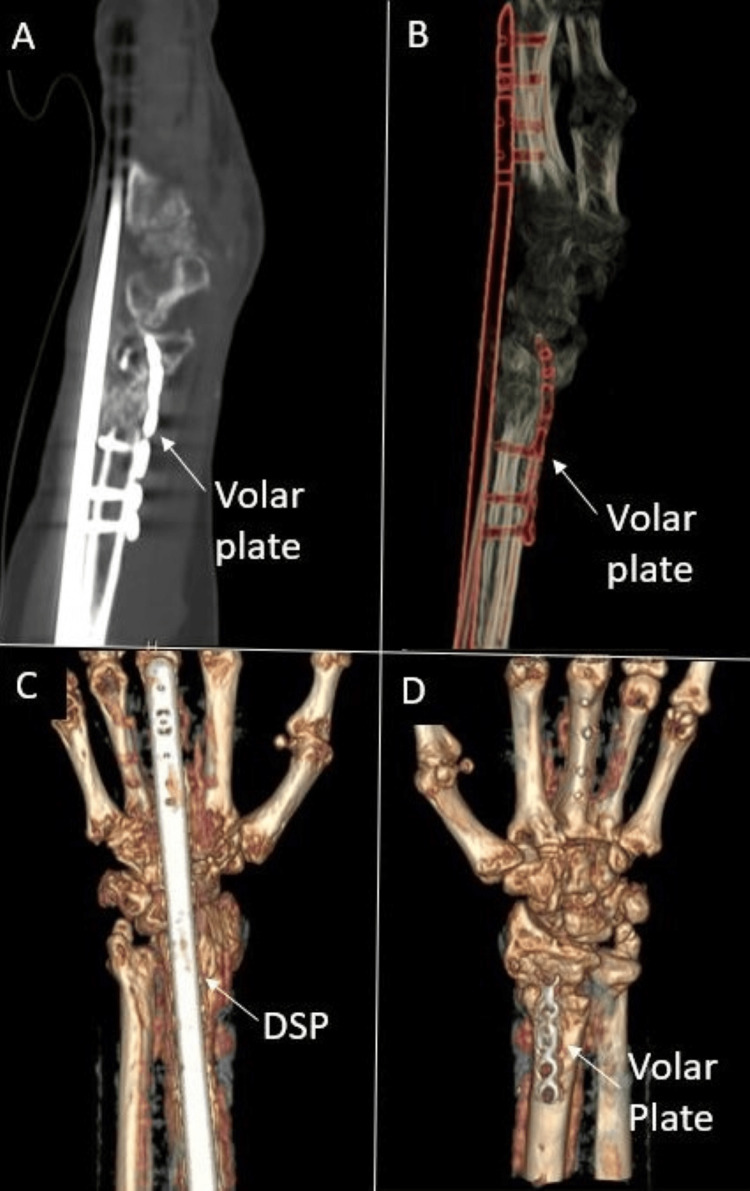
CT left wrist two months status post second fixation A/B: CT films demonstrating dorsal spanning plate (DSP) and volar intramedullary fragment plate placement C/D: 3D CT reconstruction films
